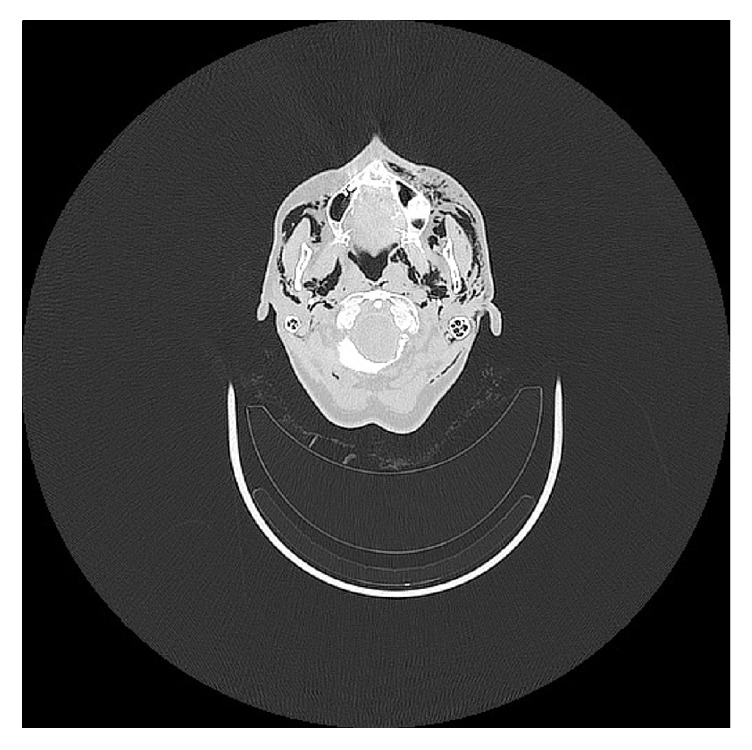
Computed tomographic axial view of the emphysema in the maxillary region.
